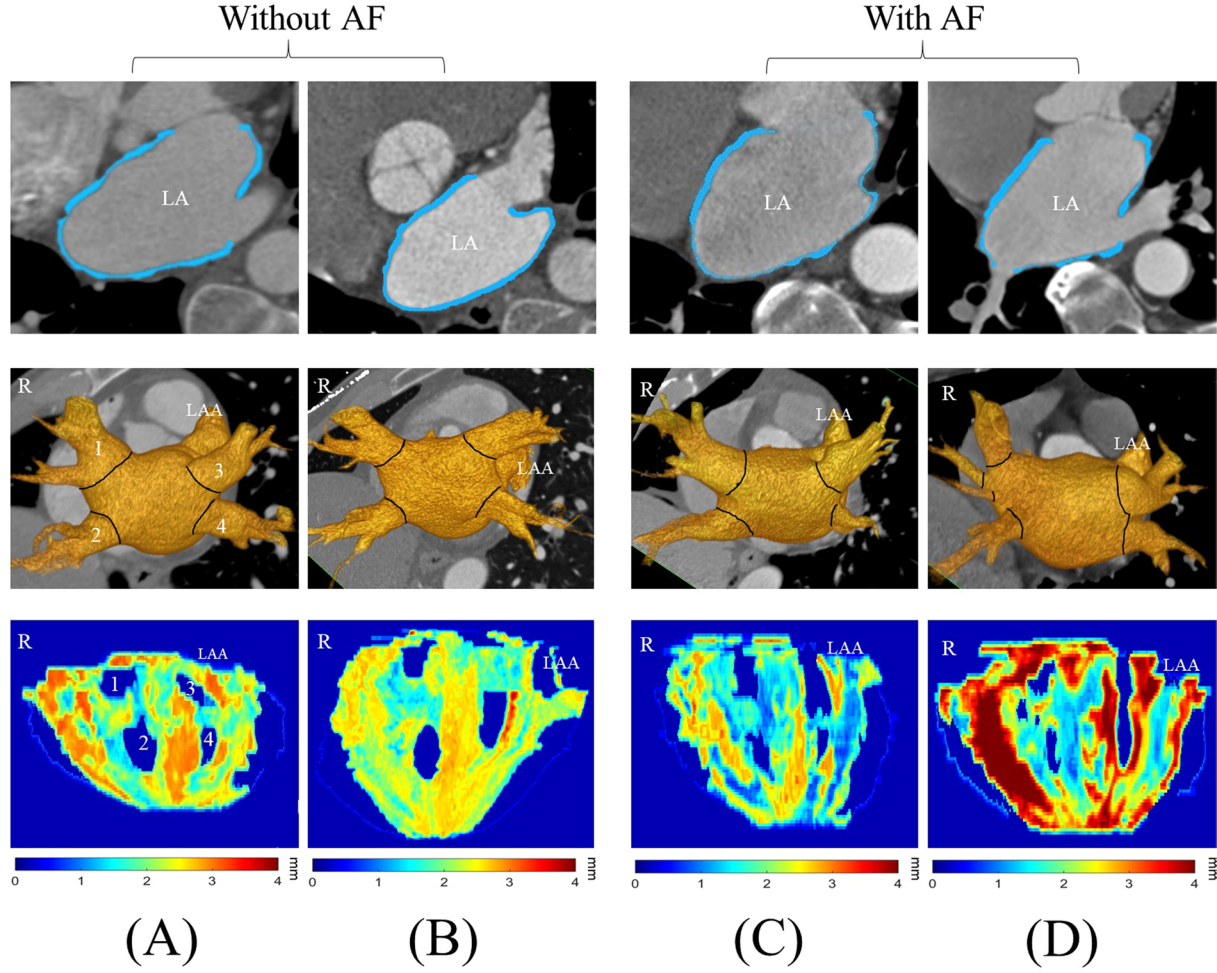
Sampleclinical results. The columns (A) and (B) show the results of the two patients without AF, and the columns (C) and (D) show the results of the two AF patients.The axial CT images, the LA surface-rendered model, and the wall map results are shown in the top, middle, and bottom row, respectively. The LA wall is marked in blue, and the black solid line represents the intersectionbetween the LA and PV. Labels 1-4 indicatethe ostium of pulmonary veins.The colour bar shows the wall thickness.
